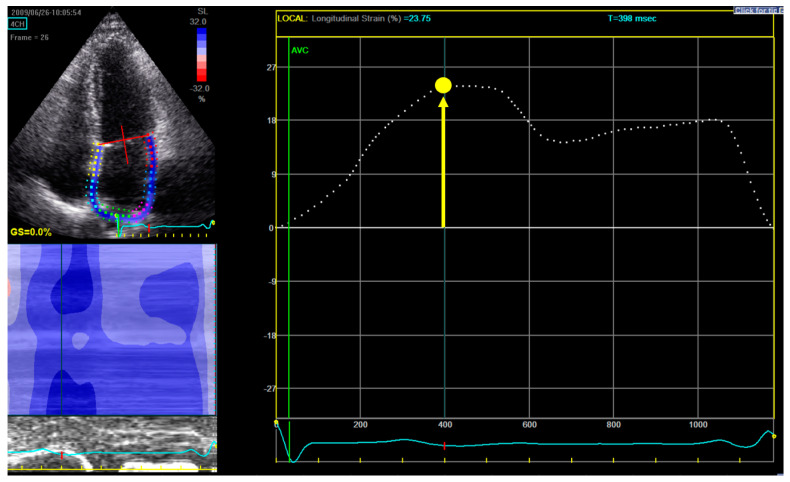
Measurement of left atrial reservoir strain (ε_R_). Upper-left panel shows the selection of the region of interest: in a dedicated non-foreshortened apical 4 chamber view, the tracing begins at the level of the endocardial border of the mitral annulus; it is then continued along the endocardial surface of the left atrium extrapolating across the orifices of the pulmonary veins and left atrial appendage, ending at the opposite side of the mitral annulus. Right panel displays the left atrium longitudinal strain curve: the zero-reference point is at end-diastole and ε_R_ is calculated as the difference between the strain value at the mitral valve opening and ventricular end-diastole (positive value). In this example in a patient from our study, ε_R_ is 23.75%.
